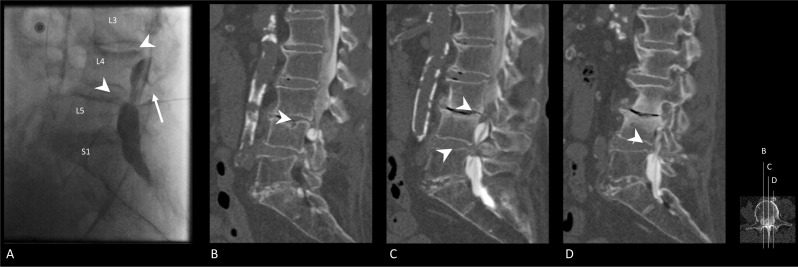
Fluoroscopic myelography and CT myelography images after lumbar punction and intradural injection with iodine-contrast medium. A Fluoroscopic myelography image after lumbar punction. The lumbar punction needle is annotated by the white arrow. Iodine-contrast is inserted into intradural space and shows spinal stenosis (white arrowheads) at level L4–L5 and, to a lesser extent, at level L3–L4. B–D Sagittal reconstructions of CT myelography (localization as depicted by the axial miniature on the right-hand side). The injected iodine-contrast is observed as radiopaque fluid in the dural sac. These images confirmed the observed spinal stenosis at level L4–L5 and L3–L4 as observed on fluoroscopic myelography. Spinal stenoses are annotated by use of the white arrowheads
